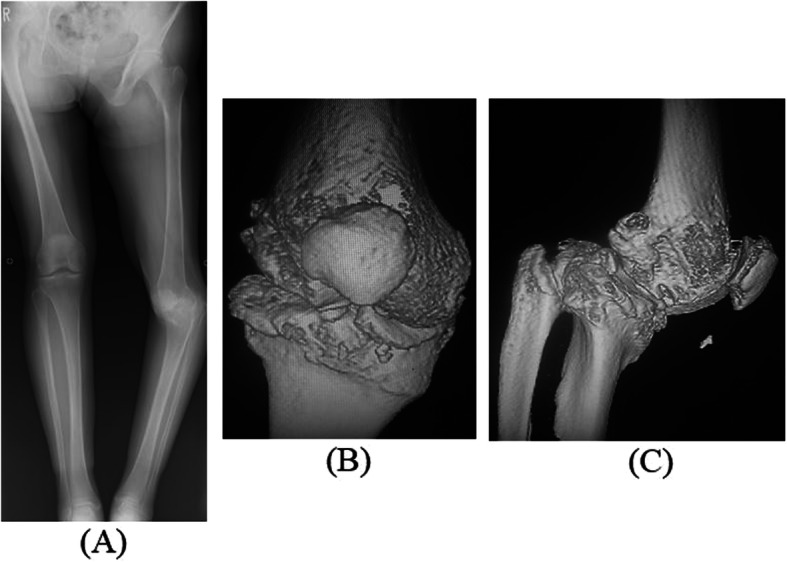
Preoperative X-ray and 3D CT. a Preoperative antero-posterior lower extremity full-length standing X-ray. b Preoperative anterior-posterior 3D CT. c Preoperative medial-lateral angle 3D CT
